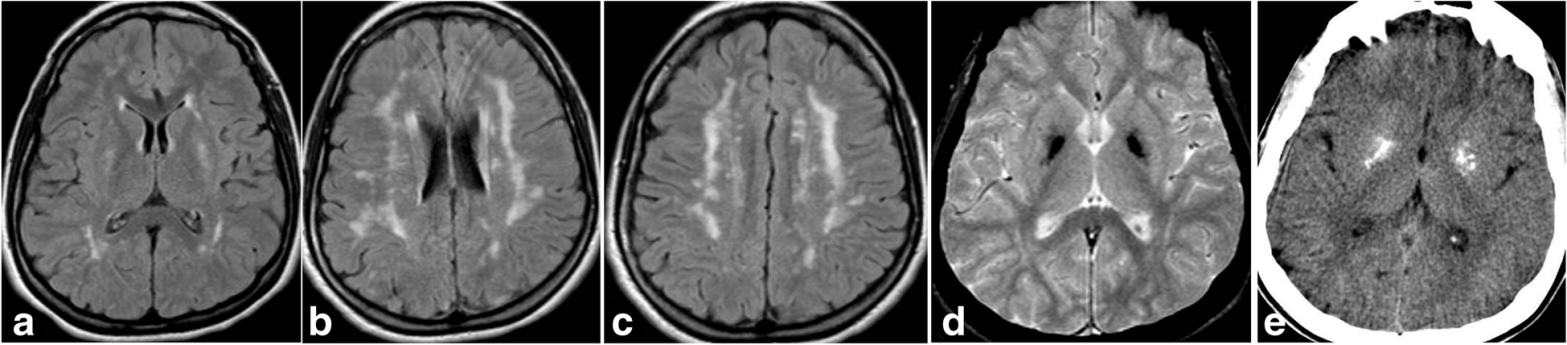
CYP2U1-related disorder- Brain MRI at 25 years old, FLAIR images in (a-c) and FFE image in (d), show bilateral hyperintensities in the putamina and confluent marked hyperintensities in the sub-cortical regions of cerebral hemispheres. In d and in e (CT), bilateral pallidal marked hypointensity and hyperdensity due to calcification is seen. Examination performed 7 years later was unchanged (data not shown)
